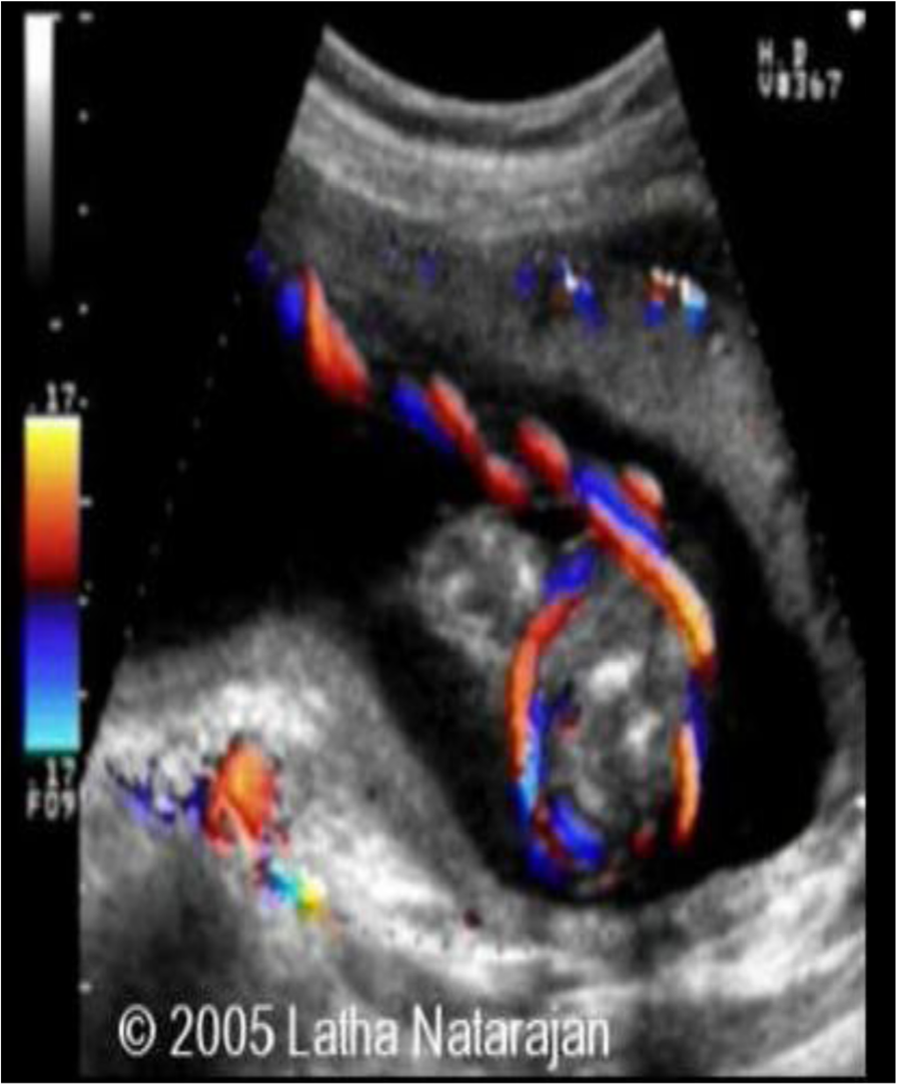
Fetal Ultra sound color doppler scan of Nuchal Cord (Courtesy from Dr. Latha Natarajan, with permission)
